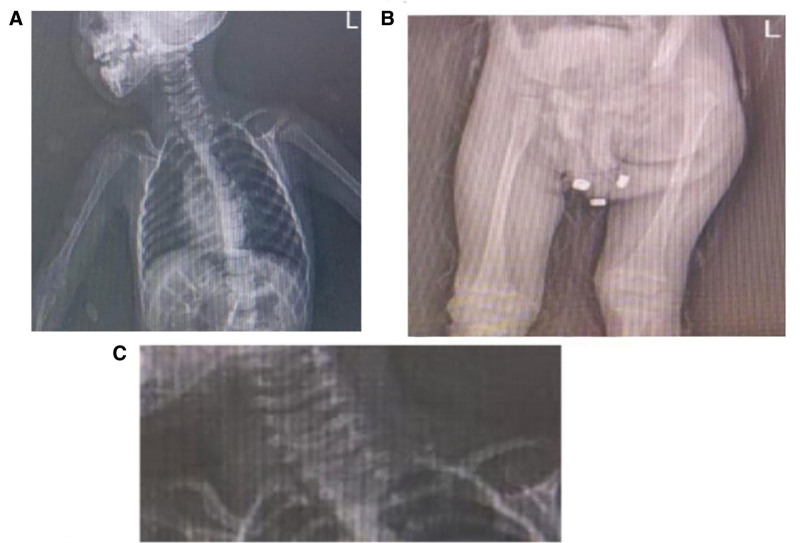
(A) Upper torso and shoulder X-ray showing malformed clavicle, elevated right scapula, and delayed ossification of ribs and vertebrae. (B) Pelvic imaging demonstrates DDH, with misalignment of the femoral head and acetabulum. (C) Lateral cervical spine X-ray reveals congenital muscular torticollis with misalignment of C1 and C2 vertebrae, suggestive of neuromuscular imbalance. DDH = developmental dysplasia of the hip.
